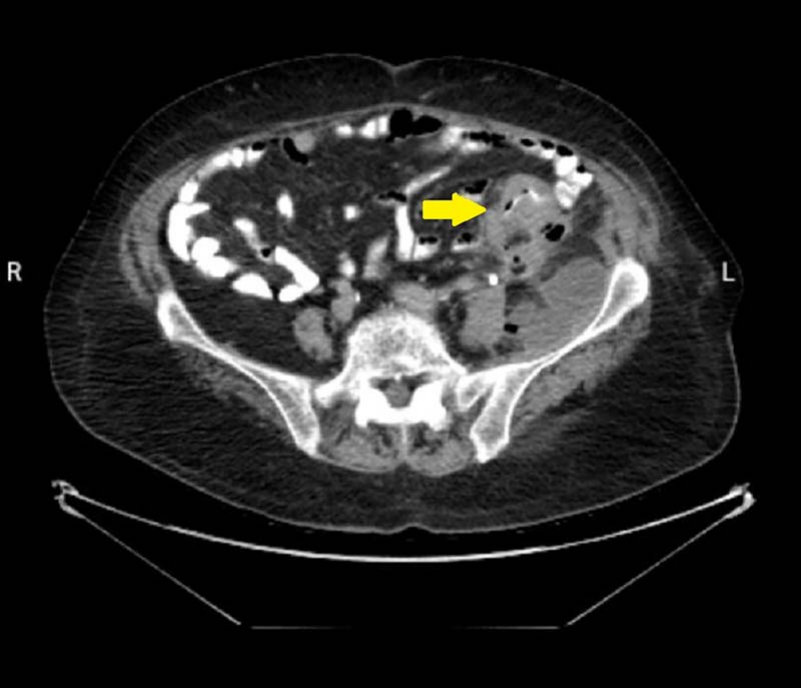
Abdominal CT scan with contrast showed the neoplasm and the presence of fluid collection (done at the time of the diagnosis of CC).
